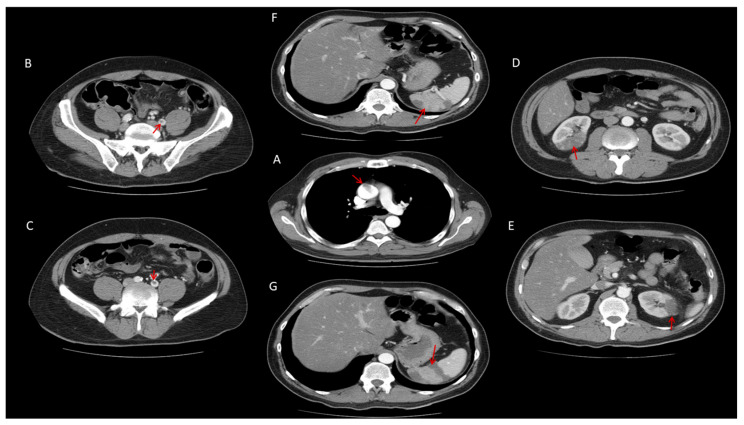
Contrast-enhanced CT of the thorax and abdomen (Day 4). (A) Mural thrombus in the ascending aorta. (B,C) Filling defects in the left iliac artery consistent with arterial thrombi. (D,E) Infarct zones in both kidneys. (F,G) Infarct zones in the liver and spleen.
